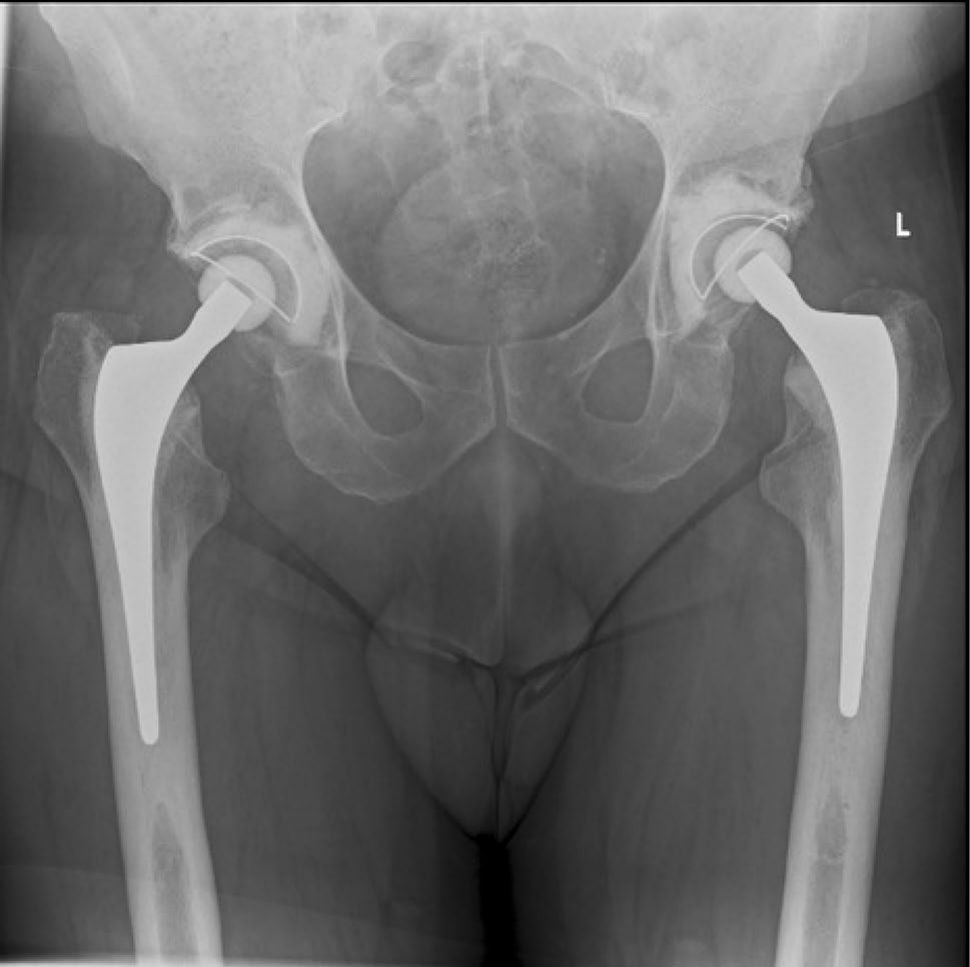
15-year follow-up radiographs for a patient that underwent staged bilateral THR for AVN at the age of 39, with primary autogenous acetabular impaction grafting performed due to marked cyst formation and sclerosis
